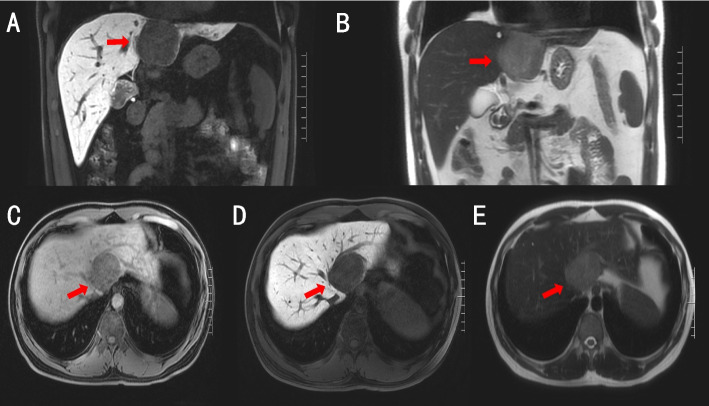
A Coronal T2-weighted MRI images (arrow indicates the lesion in segment). B Coronal T1-weighted MRI images (arrow indicates the lesion in segment). C Sagittal-plane contrast-enhanced T2-weighted arterial phase MRI images (arrow indicates the lesion in segment). D Sagittal-plane contrast-enhanced T2-weighted venous phase MRI images (arrow indicates the lesion in segment). E Sagittal-plane contrast-enhanced T1-weighted MRI images (arrow indicates the lesion in segment)
